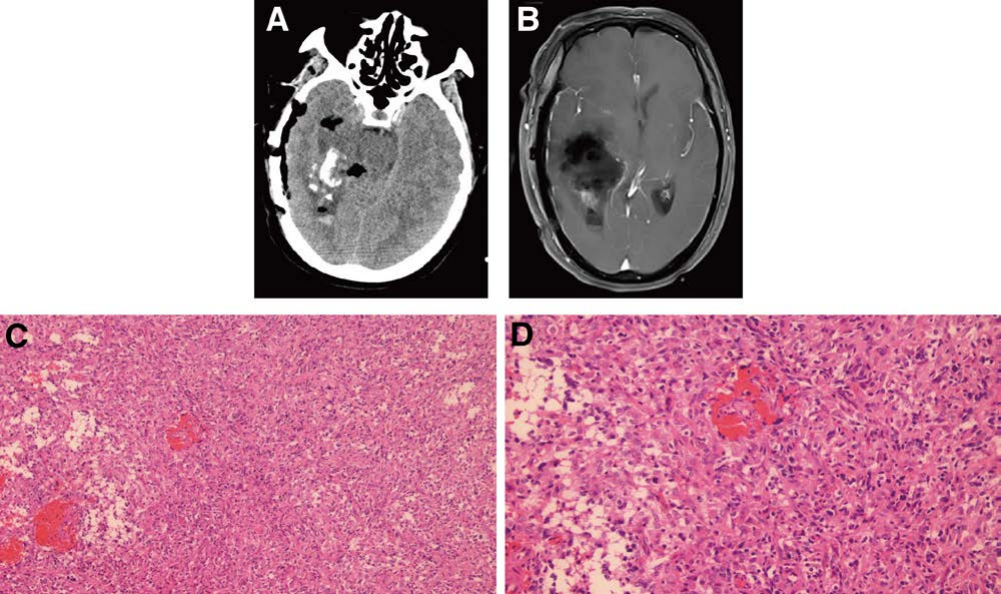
Clinical data of patient number 2. (A) Postoperative operative CT shows residual punctate and patchy calcification foci. (B) Postoperative MRI shows residual patchy enhancing lesions. (C, D) Pathology shows: tumor composed of gemistocytic astrocytes, with increased cellularity and marked atypia; microvascular proliferation is observed, with no definitive tumor necrosis (left, ×100). Gemistocytic astrocytes exhibit significant atypia, with visible mitotic figures and microvascular proliferation; no definitive tumor necrosis is observed (right, ×200). CT = computerized tomography, MRI = magnetic resonance imaging.
